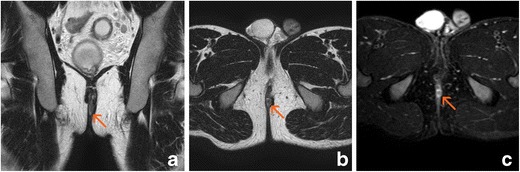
Cutaneous flap showing hyperintensity on T2-weighted images and a slight post-gadolinium rim enhancement (arrows). a Oblique-coronal T2-weighted image. b Oblique-transverse T2-weighted image. c Post-gadolinium oblique-transverse fat-supressed gradient echo T1-weighted image
